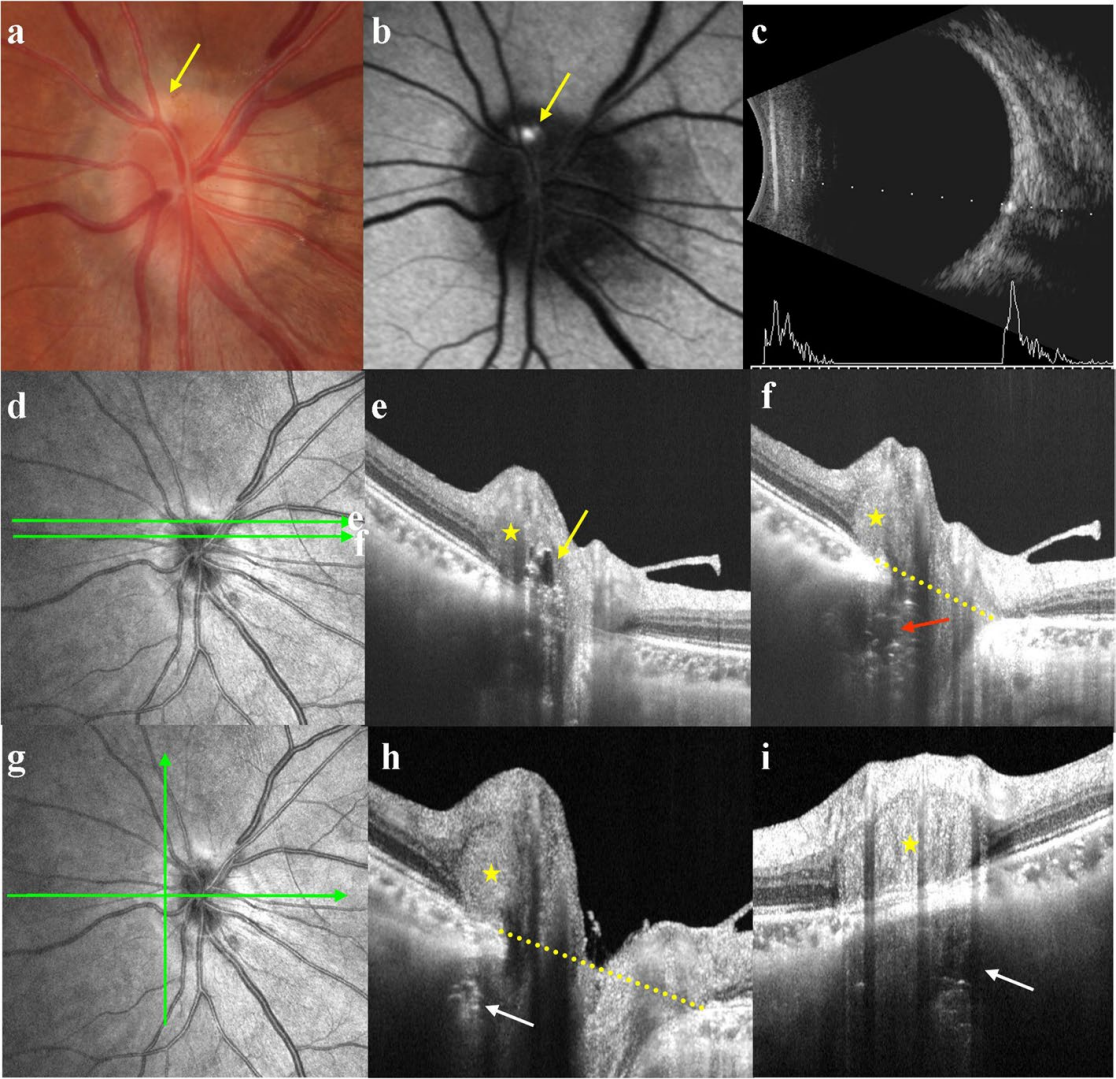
Multimodal imaging of a typical superficial ODD a CFP showed pseudopapilledema and a punctate pale-yellow deposits (yellow arrow). b FAF clearly showed autofluorescence. c: B-scan ultrasonography displayed strong echo spots with acoustic shadowing. d The upper scan line corresponded to e and the lower scan line corresponded to f. e The ODD was in the neuroepithelial layer of optic disc nasal superior (yellow arrow corresponding to yellow arrow in a and b). f The ODD was near the center of the optic disc (red arrow). g Horizontal scan line corresponded to h, vertical scan line corresponded to i. h and i ODD was in the border of Elschnig. The yellow stars in e, f, h and i indicated PHOMS, corresponding to pseudopapilledema. The yellow dotted line in f, h indicated the BMO. CFP = color fundus photography; FAF = fundus autofluorescence; PHOMS = peripapillary hyperreflective ovoid mass-like structure; BMO = Bruch’s membrane opening
